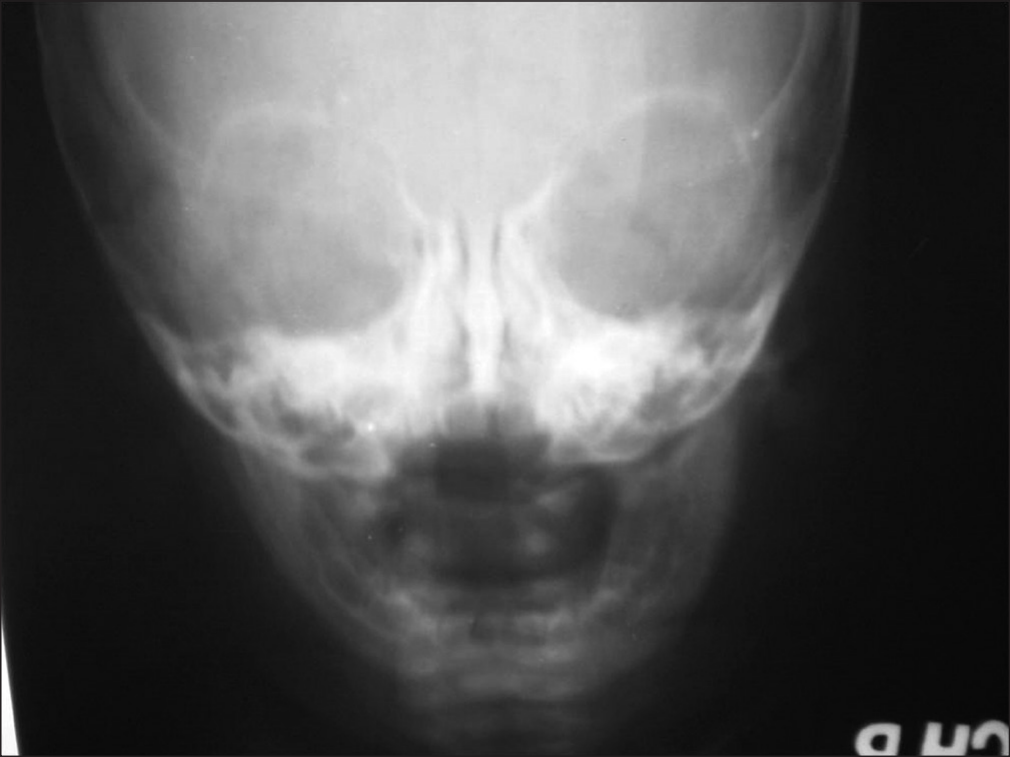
X-ray of the skull showing diffuse thickening and increased density of maxilla and mandibles
